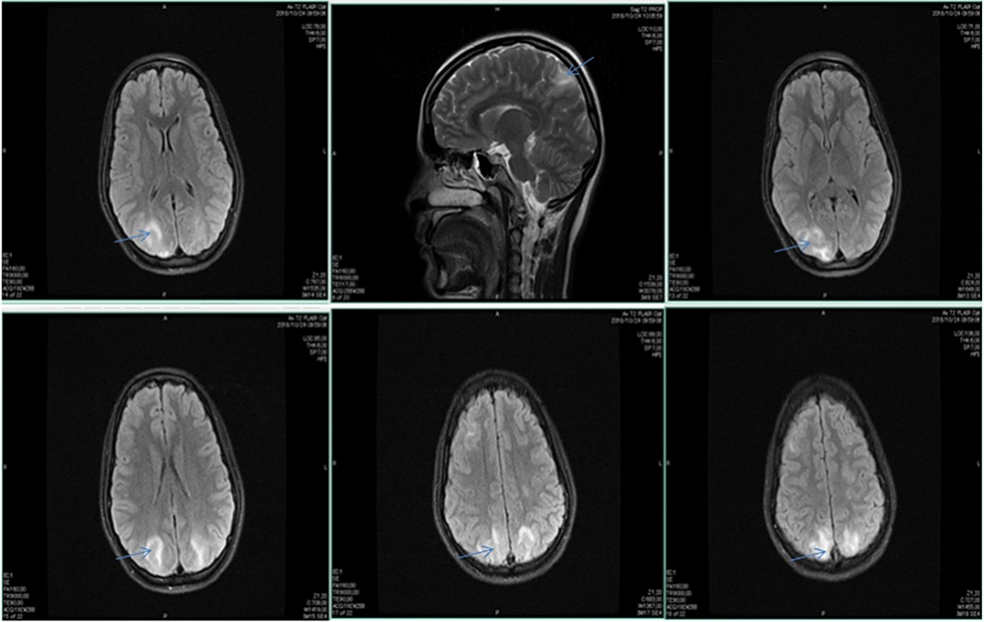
Cerebral MRI T2-weighted spin-echo and gradient MRI sequences in axial and sagittal planes, with subcortical T2 signal enhancement in the posterior temporal and occipital regions indicated with straight arrows. This signal increase is compatible with edema, predominantly vasogenic. In T2 gradient sequences, there was no evidence of hemorrhagic lesions MRI: magnetic resonance imaging
